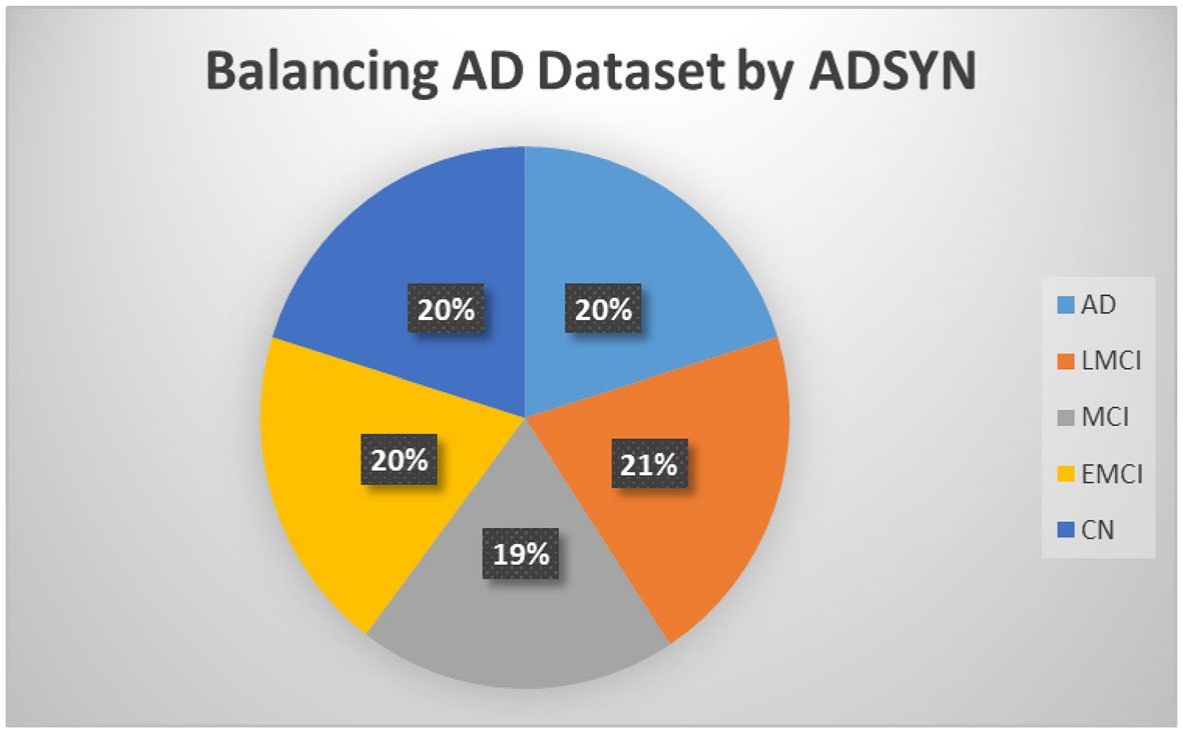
Class distribution of the MRI dataset after oversampling.
